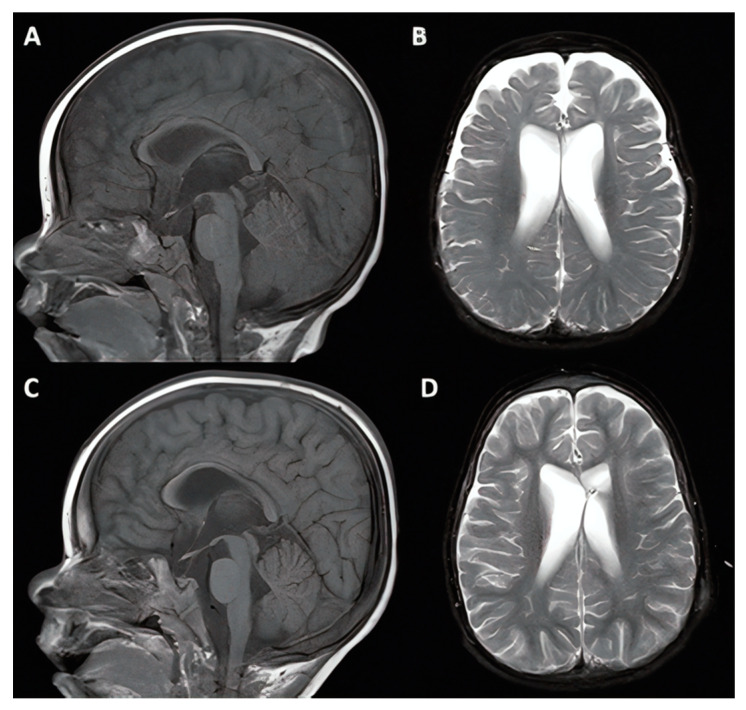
17-month-old male patient with cerebral palsy (case #2). Pre-operative (A,B) and 1 year post-operative (C,D) T1-weighted midsagittal and T2-weighted axial magnetic resonance imaging (MRI).
